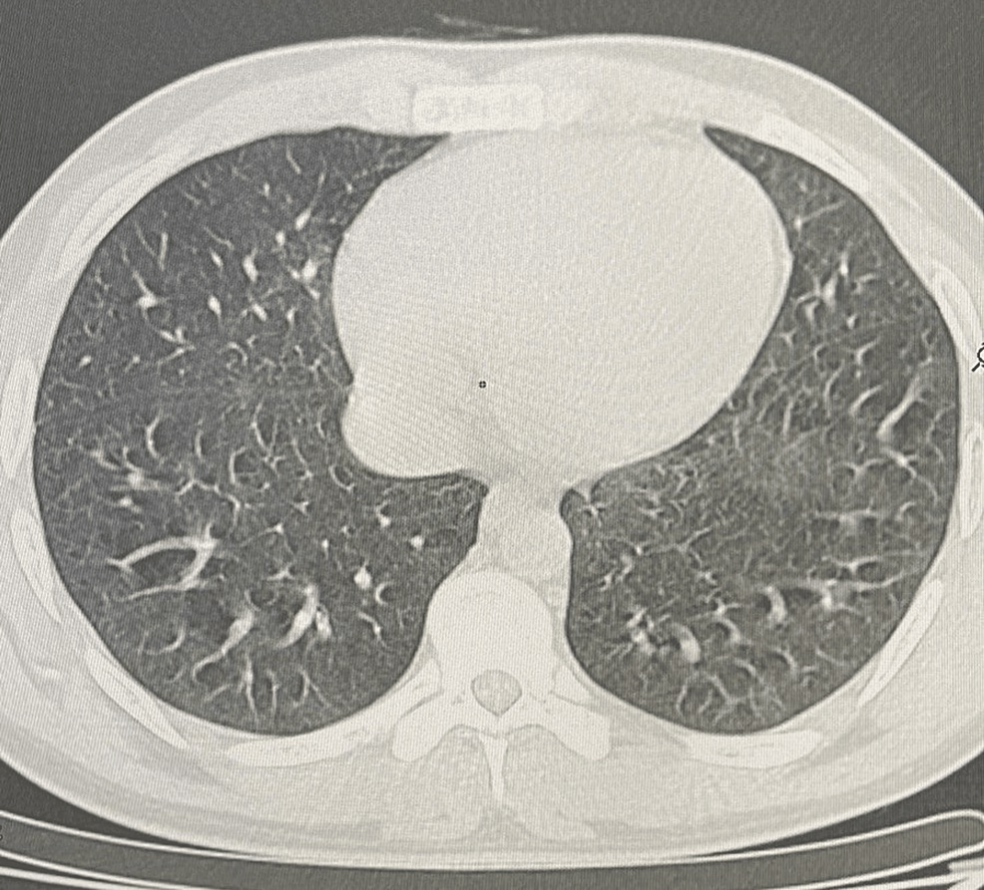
Scannographic clean up Thoracic CT scan showing absence of progressive pleuropulmonary lesions
